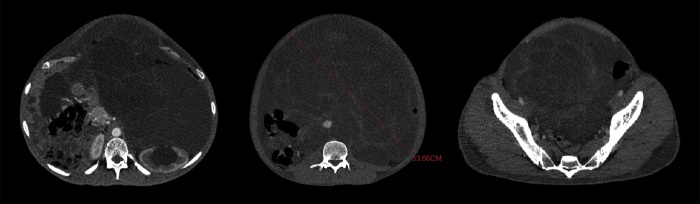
The CT scan that was made before the first operation: showing the huge extension of the cyst occupying all the abdomen, pushing other abdominal organs to the right.
